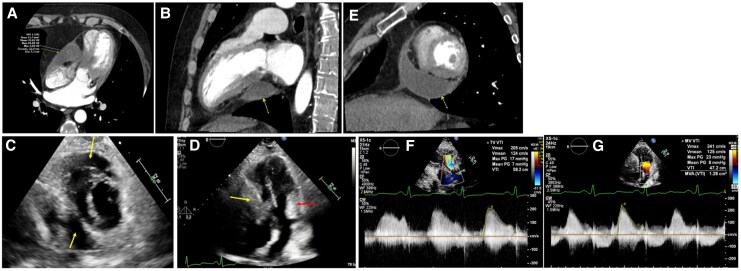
Cardiac CTA demonstrated a loculated pericardial effusion (arrows) exerting mass effect on the right atrium and ventricle, and to a lesser degree the left-sided chambers, with mean attenuation of 36 Hounsfield units suggesting a non-simple fluid collection (panels *A*, *B*, *E*). TTE confirmed chamber compression by the echolucent pericardial mass (arrows) with respiratory septal shift indicating ventricular interdependence, and additional compression of the mitral inflow near the lateral annulus (arrow, Panels *C*, *D*). Continuous-wave Doppler revealed elevated velocities and mean diastolic gradients across the tricuspid (mean diastolic gradient 7 mmHg at 75 bpm) and mitral valves (mean diastolic gradient 8 mmHg at 75 bpm), consistent with extrinsic compression from the pericardial mass (Panels *F*, *G*).
